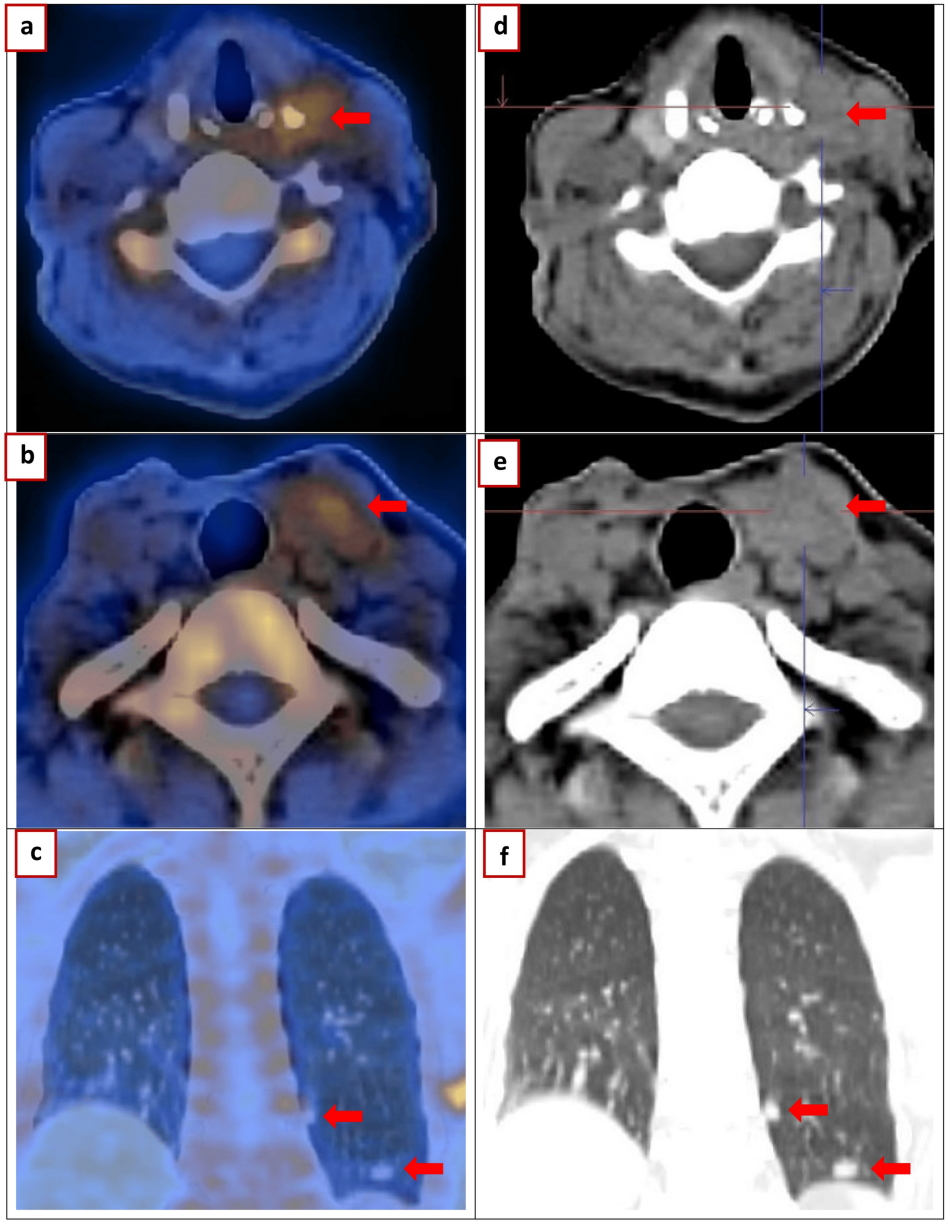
Nuclear medicine imaging (a-f) 2024 May: Gallium (Ga)-fibroblast activation protein inhibitor (FAPI) PET/CT (fibroblast activating protein; Oncoview). Ga-FAPI positron emission computed tomography imaging demonstrated high expression of fibroblast activation protein (FAP) in all tumor sites. Soft tissue nodules in the left thyroid bed and adjacent mid jugular region standardized uptake value (SUV): 1.7 (largest 15 × 11 mm; progression); left lower cervical and supraclavicular lymph nodes (largest 6 × 5 mm; regression); left lung nodules SUV: 0.8 (9 mm; additional bilateral nodules up to 9 mm; progression).
